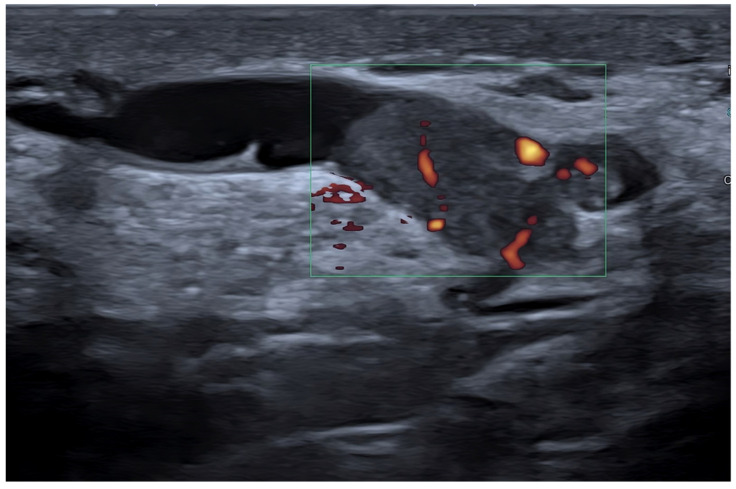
Breast intraductal papilloma. Detailed morpho-structural assessment working with a high-frequency transducer (22 MHz). Power Doppler detection of tumor vessels.
